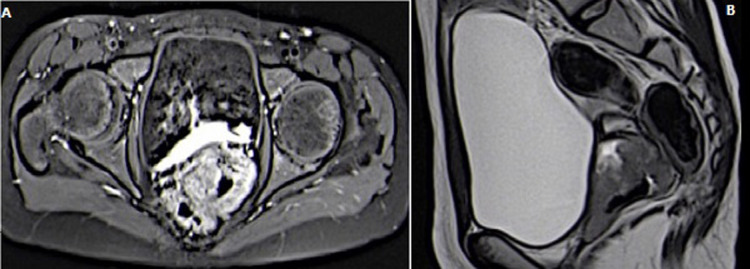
A) T1 FAT SAT with contrast MRI shows mass occupying the vagina with heterogeneous enhancement; B) T2 without contrast showing the vaginal mass with cervical involvement and respect of posterior side of the bladder
